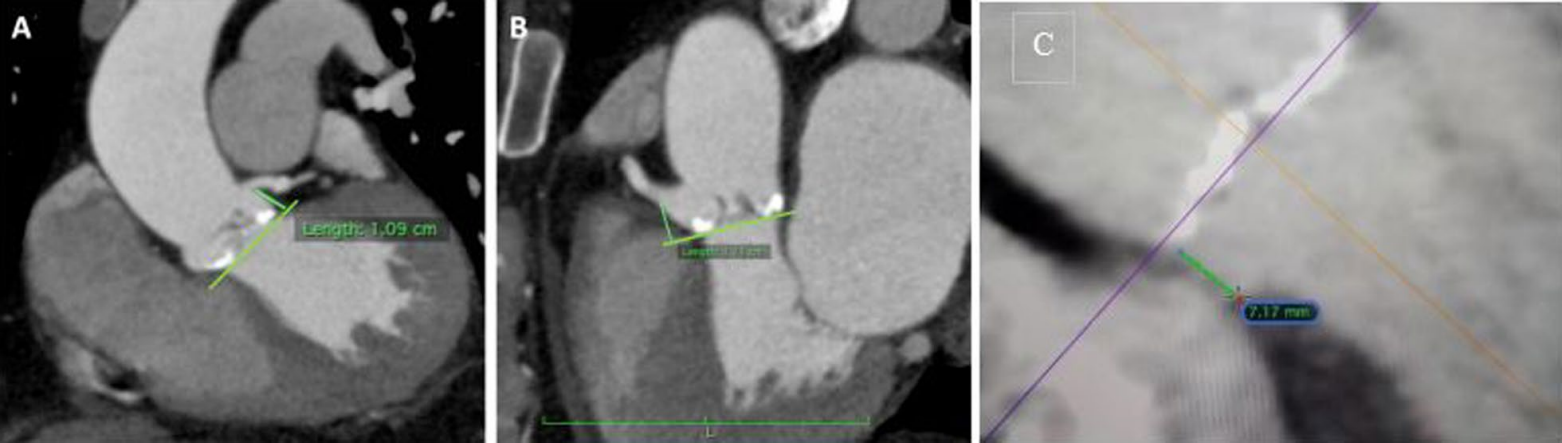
CT-derived measurements of heights of the coronary arteries Ostia from the aortic annulus and membranous septum length
